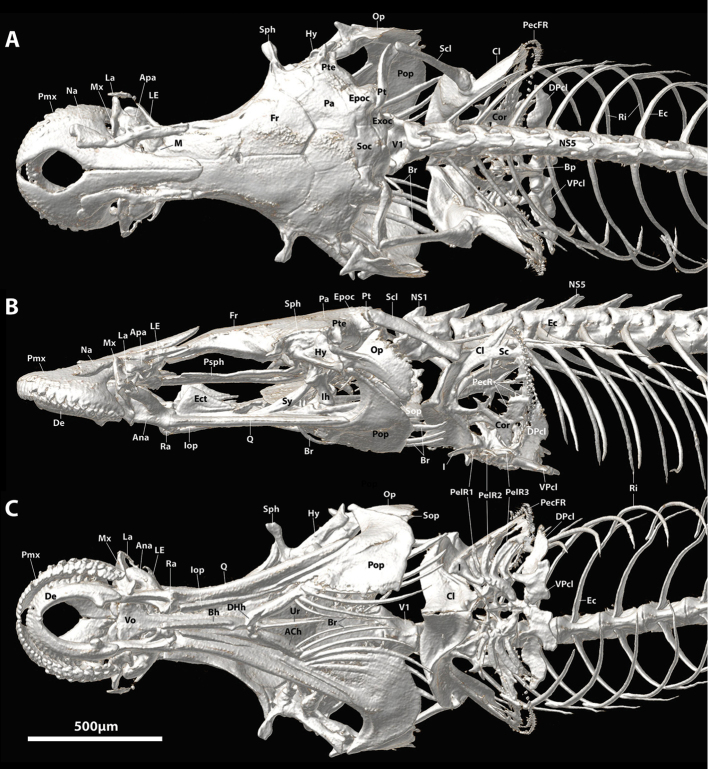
CT scanned anterior skeleton of *Lepadichthysbolini*, ROM 55185, 21.5 mm SL. A Dorsal view B Lateral view (left side) C Ventral view. Abbreviations: ACh, anterior ceratohyal; Ana, anguloarticular; Apa, autopalatine; Bp, basipterygium; Br, branchiostegal rays; Cb5, ceratobranchial 5; Cl, cleithrum; Cor, coracoid; DHh, dorsal hypohyal; DPcl, dorsal postcleithrum; Ec, epicentral; Ect, ectopterygoid; Epoc, epiotic; Exoc, exoccipital; Fr, frontal; Hy, hyomandibular; I, pelvic-fin spine; Iop, interopercle; La, lachrymal; LE, lateral ethmoid; M, mesethmoid; Na, nasal; NS1, 5, neural spine of vertebral centrum 1, 5; Op, opercle; Pa, parietal; PecR, pectoral radial; PecFR, pectoral-fin ray; PelFR1-4, pelvic-fin ray 1–4; Pop, preopercle; Pro, prootic; Psph, parasphenoid; Pt, posttemporal; Pte, pterotic; Q, quadrate; Ra, retroarticular; Ri, rib; Sc, scapula; Scl, supracleithrum; Soc, supraoccipital; Sop, subopercle; Sph, sphenotic; Ur, urohyal; V1, vertebral centrum 1; Vo, vomer; VPcl, ventral postcleithrum.
